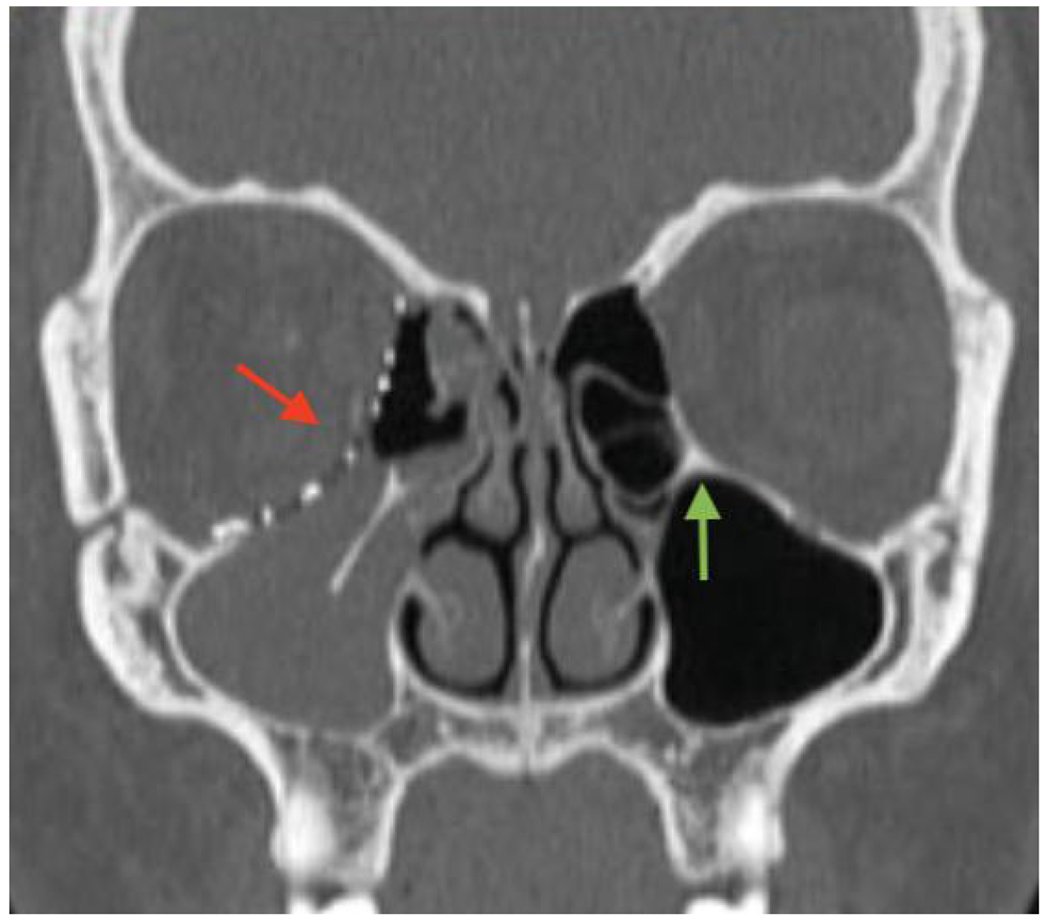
A 31-year-old woman sustained fractures of the right orbital floor and medial wall. Postoperative CT scan demonstrating the absence of internal orbital buttress requiring placement of a single, large implant (red arrow) covering both fractured walls, and intact buttress on contralateral unfractured side (green arrow). (Re-published from Nguyen *et at.* with permission from SAGE Publishing^[8]^).
